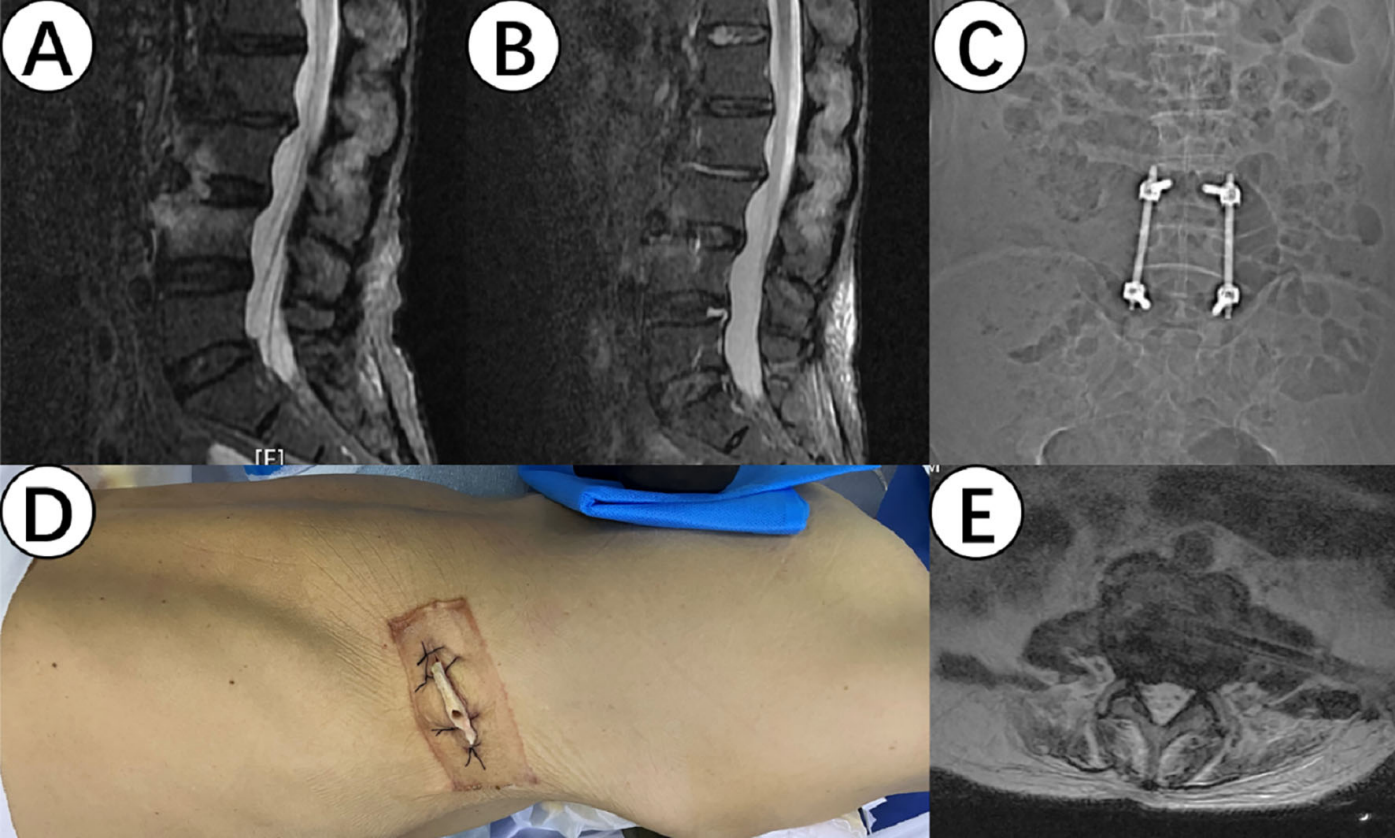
(A, B) show the magnetic resonance imaging (MRI) results of the lesion site before and after surgery, demonstrating a significant improvement in inflammatory signals. (C) shows internal fixation for intersegmental fixation to prevent lumbar instability and collapse. (D) shows the implantation of a drainage sponge through an extremely lateral approach. (E) shows the position of the drainage sponge in the intervertebral gap on the MRI.
